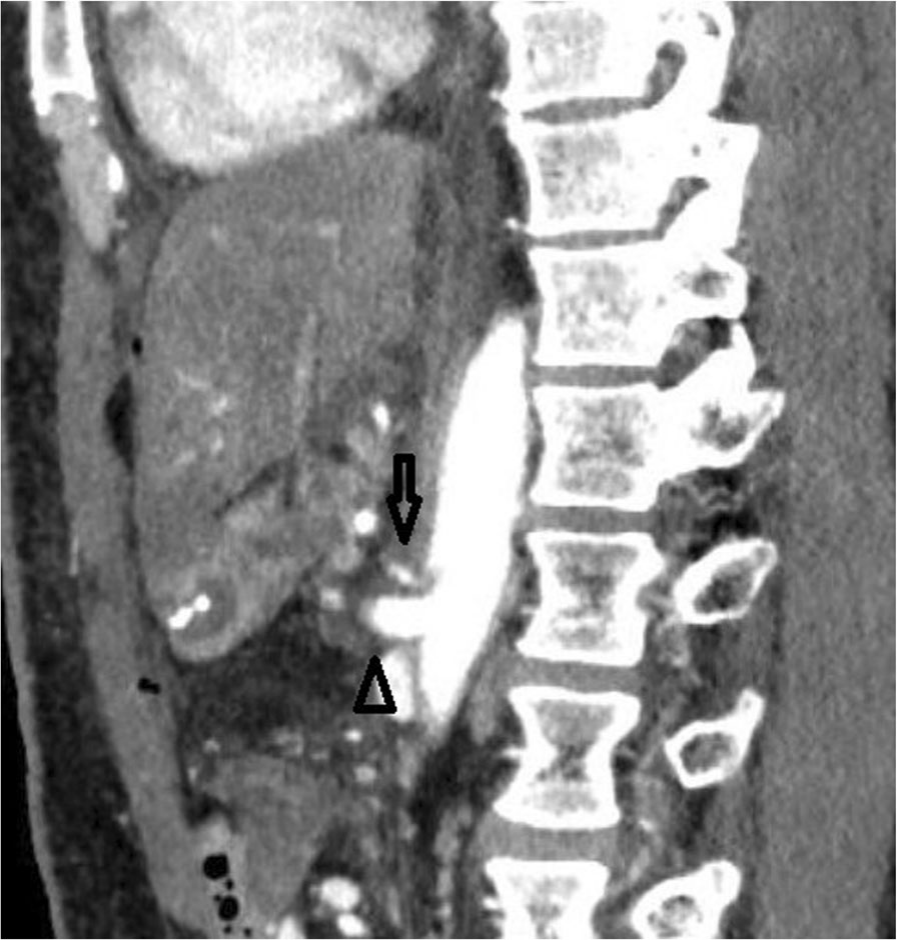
Preoperative multidetector CT with routine arterial reconstruction showing normal celiac artery anatomy and no evidence of median arcuate ligament syndrome (the *arrow* shows the CA and the *arrow head* shows the SMA)
